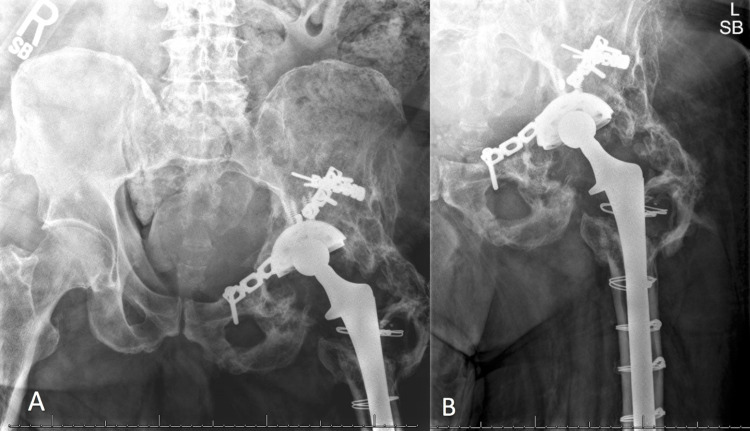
Preoperative radiographs of the pelvis. Preoperative anterior-posterior radiograph of the pelvis (a) and anterior-posterior radiograph of the left hip (b) demonstrating acetabulum hardware with broken plate, prior left total hip arthroplasty, heterotopic ossification, osteolysis, and large colonic stool burden.
